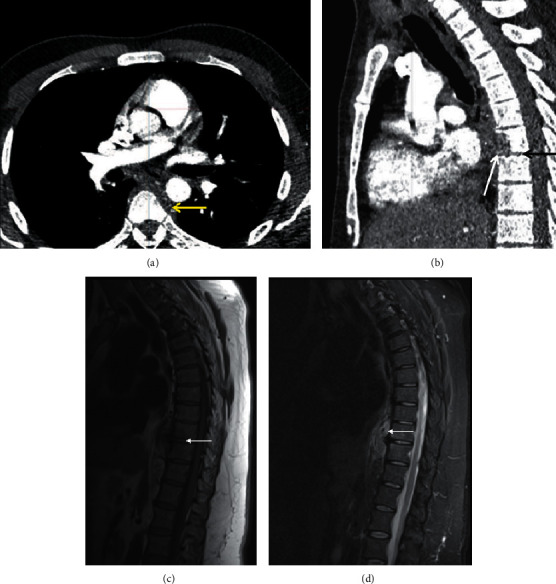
Tuberculous osteomyelitis. Postcontrast axial (a) and sagittal (b) chest CT of a 34-year-old patient with biopsy-proven TB discitis-osteomyelitis. There is lytic destruction of the T7 vertebral body anteriorly ((b) white arrow). There is subligamentous soft tissue density extending from T5-T8 (A yellow and B white arrows) with relative sparing of the adjacent T7 end plates (b black arrow). Sagittal T1 (c) and STIR (d) of a different patient with T9/10 osteomyelitis (c arrow) and pronounced subligamentous spread of disease from T8/9-T11 (d arrow). Note the relative sparing of the disc in early disease (c arrow).
